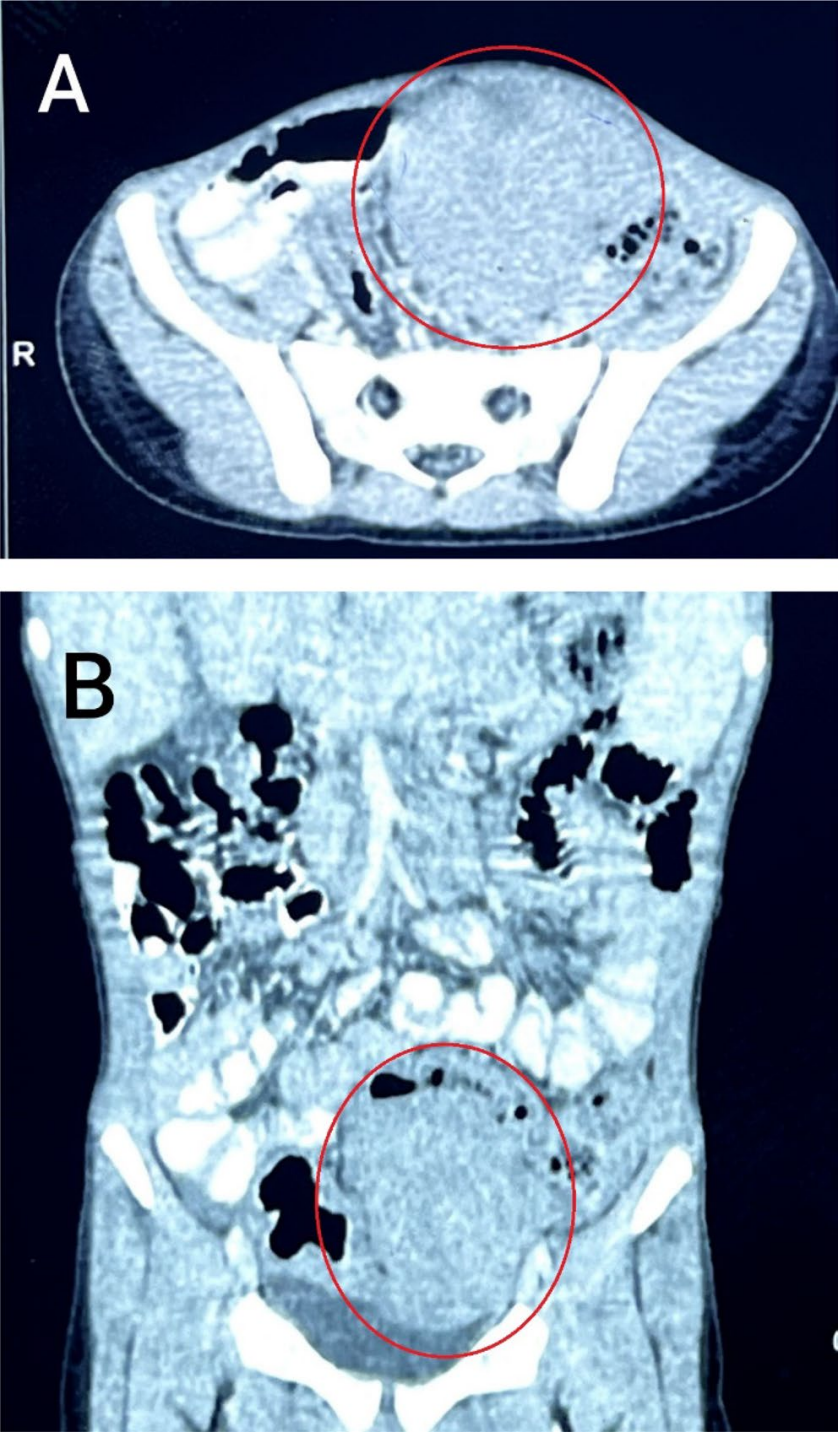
Abdominal computed tomography (CT) scan showing (A) an axial section of the abdominopelvic mass narrowing the sigmoid colon lumen and (B) a coronal section of the mass.
